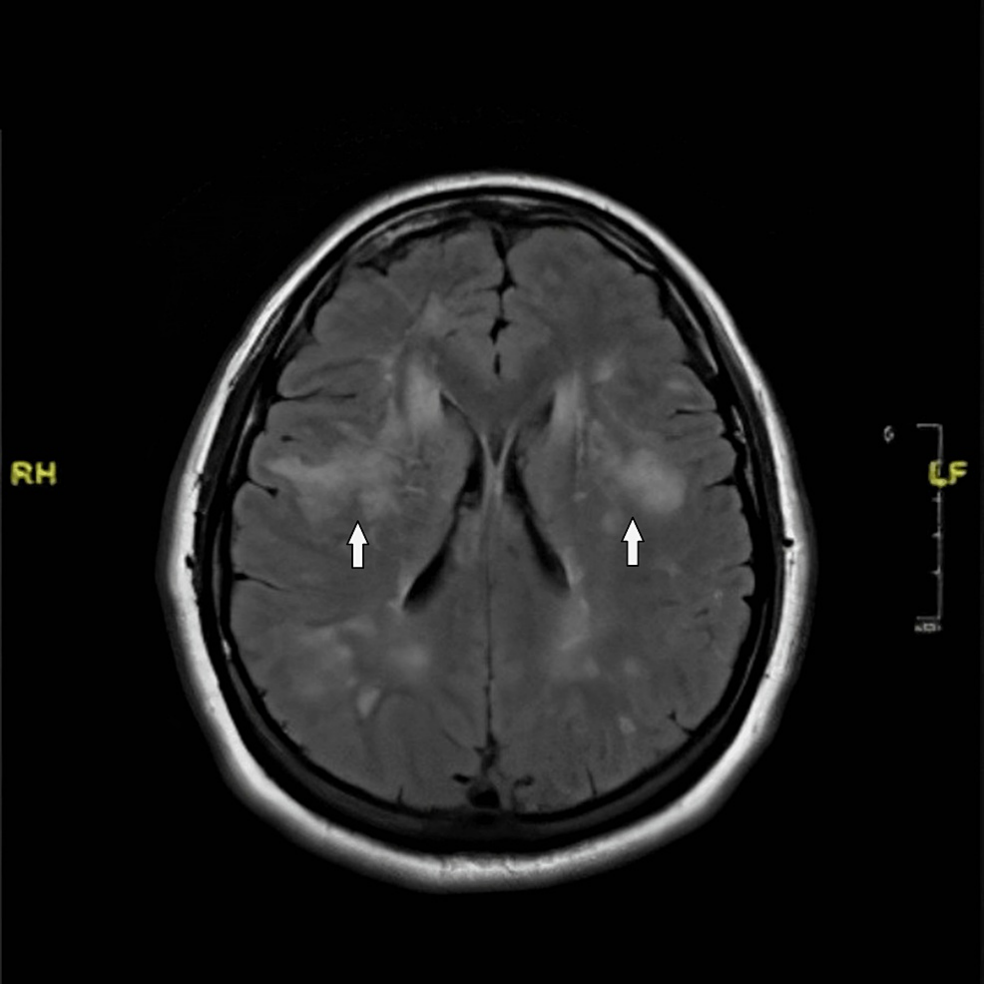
MRI of the brain (T2/FLAIR) White arrows show diffuse, disseminated, confluent, relatively symmetric white matter signal disease process involving periventricular and prominently subcortical white matter tracts MRI: magnetic resonance imaging; FLAIR: fluid-attenuated inversion recovery
